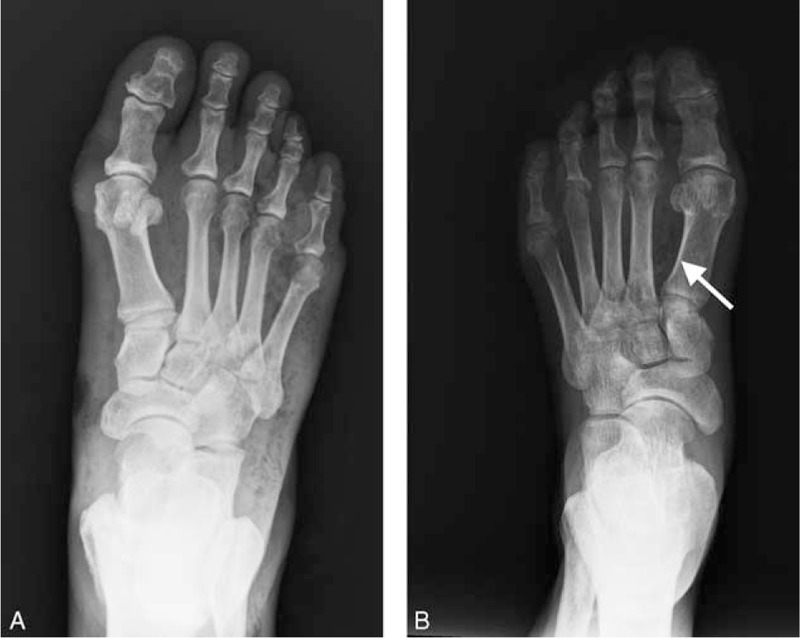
Gas formation revealed by plain radiograph in NF patients with DFU. (A) A 55-year-old male presented with 4th and 5th toes wet gangrene in the right foot. Subcutaneous emphysema was noted all over the foot. (B) A 50-year-old male presented with a grade 3 wound over plantar area of left foot. X-ray examination revealed air pockets between the big toe and 2nd toe (arrow). DFU = diabetic foot ulcer, NF = necrotizing fasciitis.
